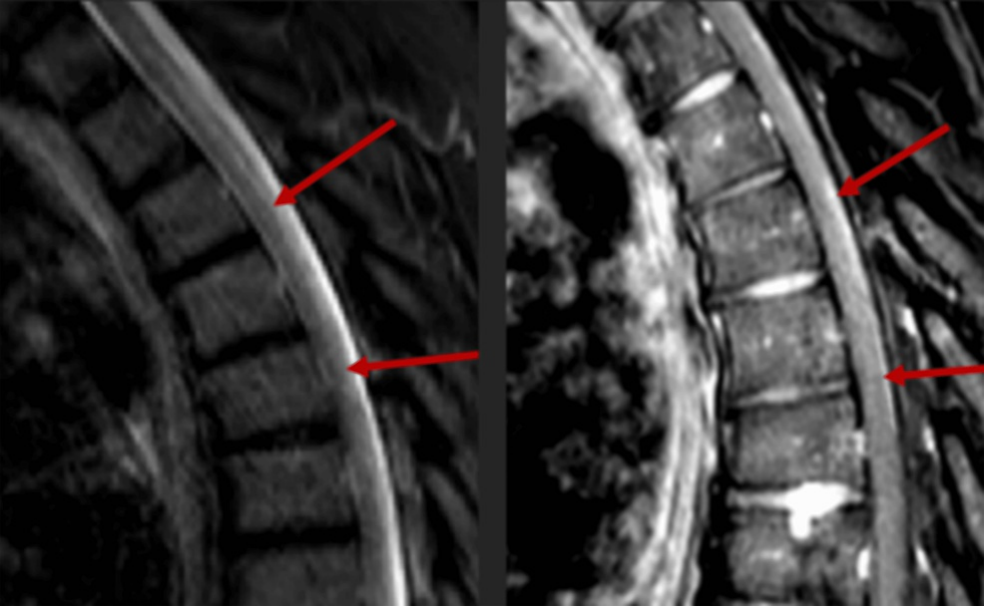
MRI of the spine showing a hyperintense signal on short tau inversion recovery (STIR) sequence (left) and gadolinium enhancement on T1-weighted image (right) indicating active inflammation in the posterior cord extending from the C7 to the T5 vertebrae (arrows). These findings are consistent with longitudinally extensive transverse myelitis.
